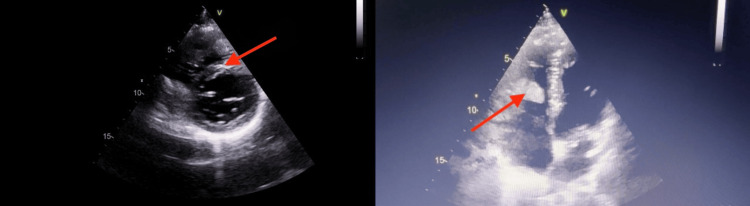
Echocardiographic images showing right ventricular thrombi in the parasternal short-axis and apical four-chamber views (arrows)
